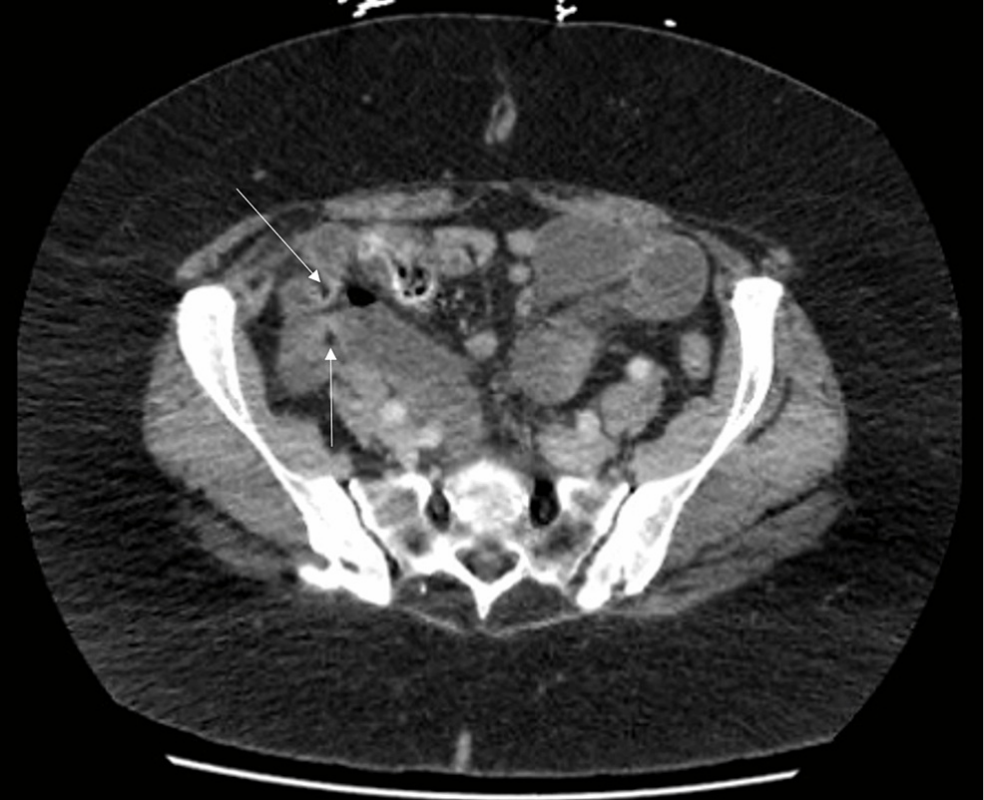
Axial view of computed tomography scan of the abdomen Arrows indicate transition points of the small bowel within pericaecal hernia.
